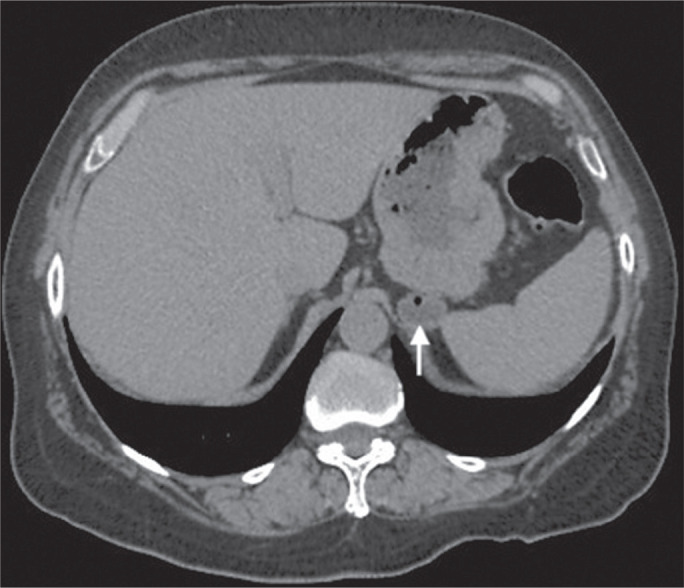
A 65-year-old female with rheumatoid arthritis. Chest CT showing a
